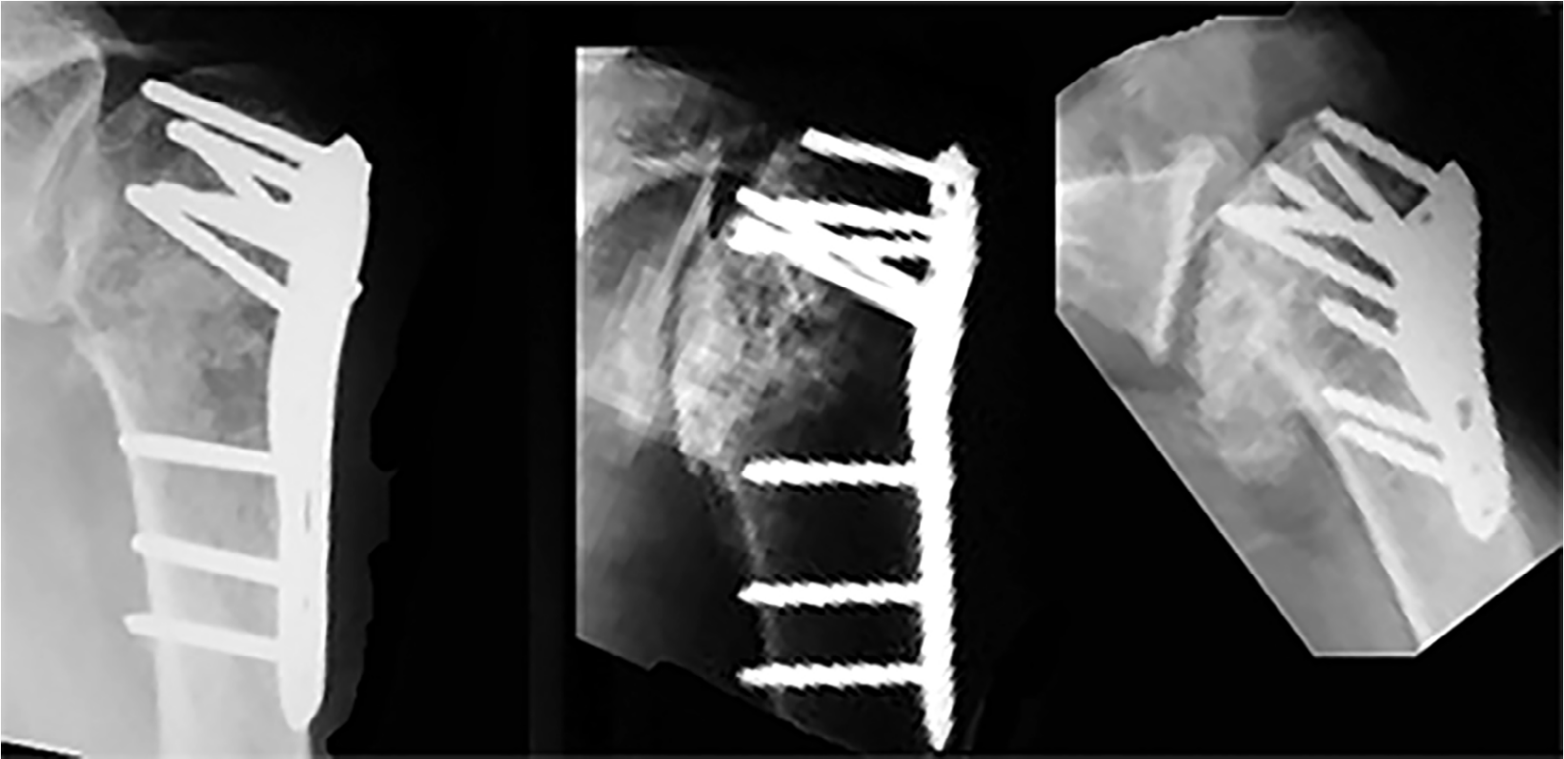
Posttraumatic radiograph of humeral head and evolution to osteonecrosis.
